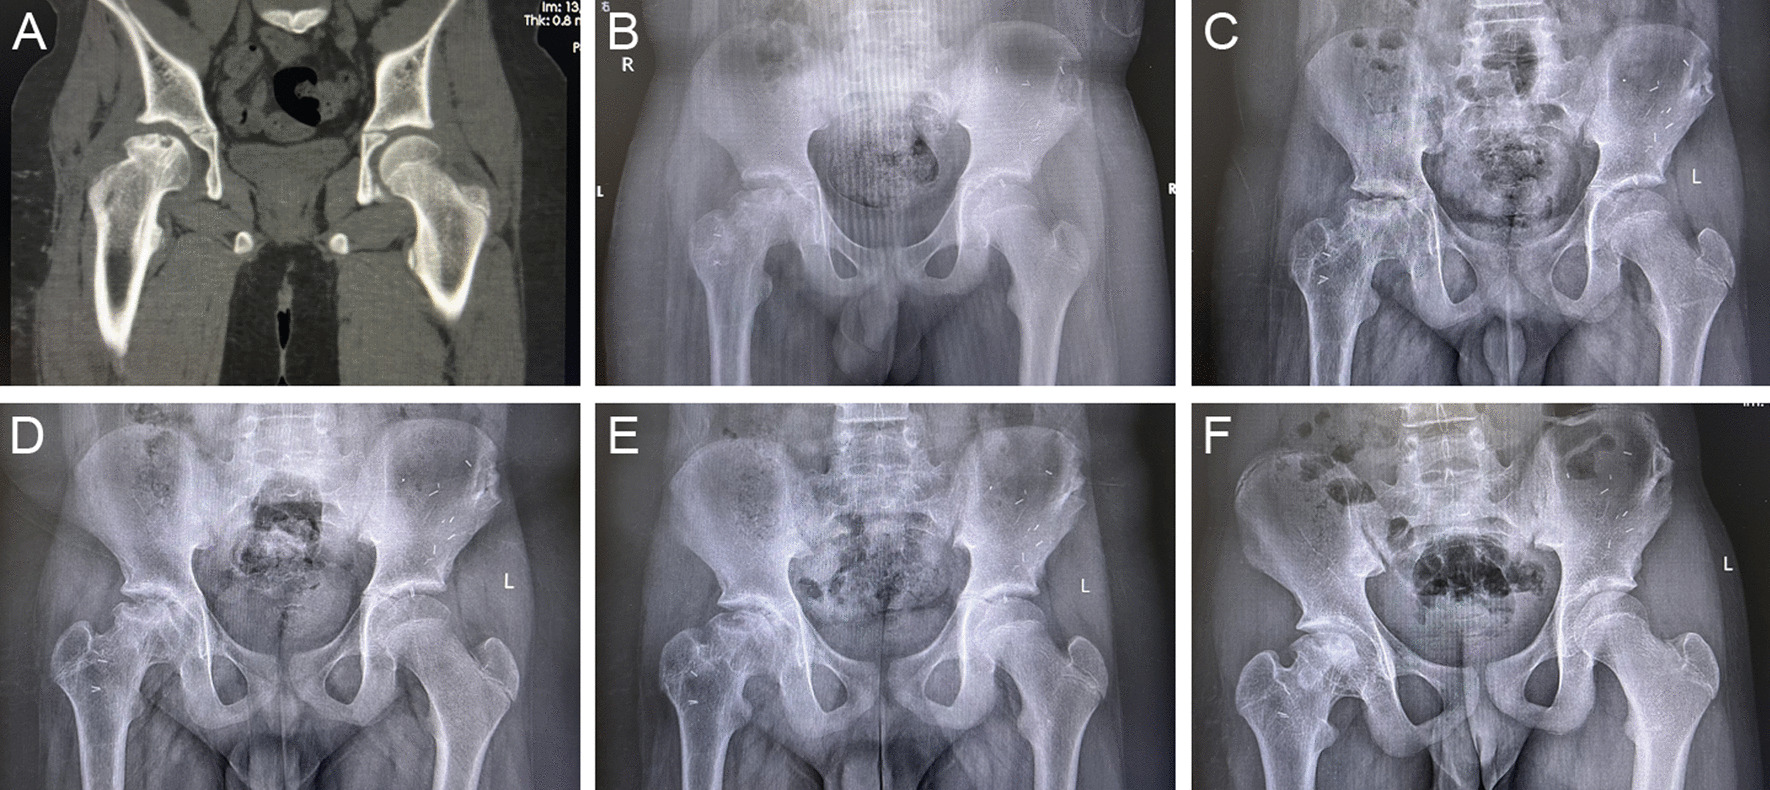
Case 1: A 11-year-old men patient with ARCO III traumatic ONFH at right received the treatment of anterograde anatomy iliac bone flap transfer. A Preoperative radiograph of CT image: note the distinct flattening, clear necrosis and osteolysis. B–F The anteroposterior radiograph of the pelvis at 1 months, 3 months, 6 months, 12 months, 24 months postoperatively: through the whole follow-up, round shape of femoral head is protected, with no progressive collapse and a well-preserved joint space. Iliac graft incorporates with the femoral head better with prolonged follow-up
